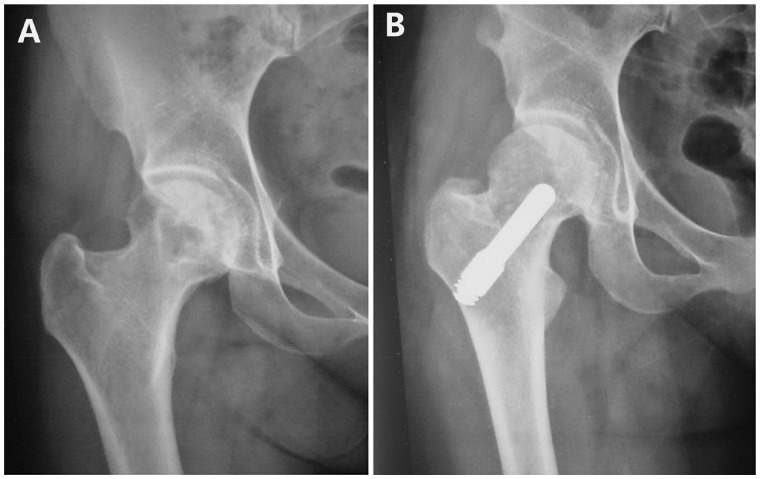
A 38-year-old female with ARCO stage III ONFH (right hip) received the treatment of tantalum rod implantation combined with vascularized iliac grafting. Radiographs were taken pre-operation (A), 36 months post-operation (B). (B) The joint space remains preserved, the iliac bone graft is well incorporated. Reprinted from Ref. [75] with permission
